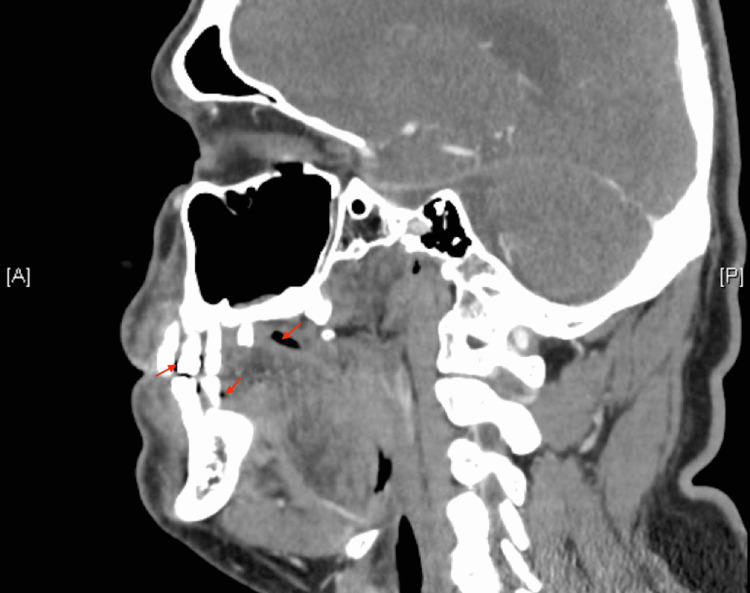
Computed tomography scan of facial bones revealing mandibular and maxillary periapical abscesses bilaterally with soft tissue thickening of the maxilla.
